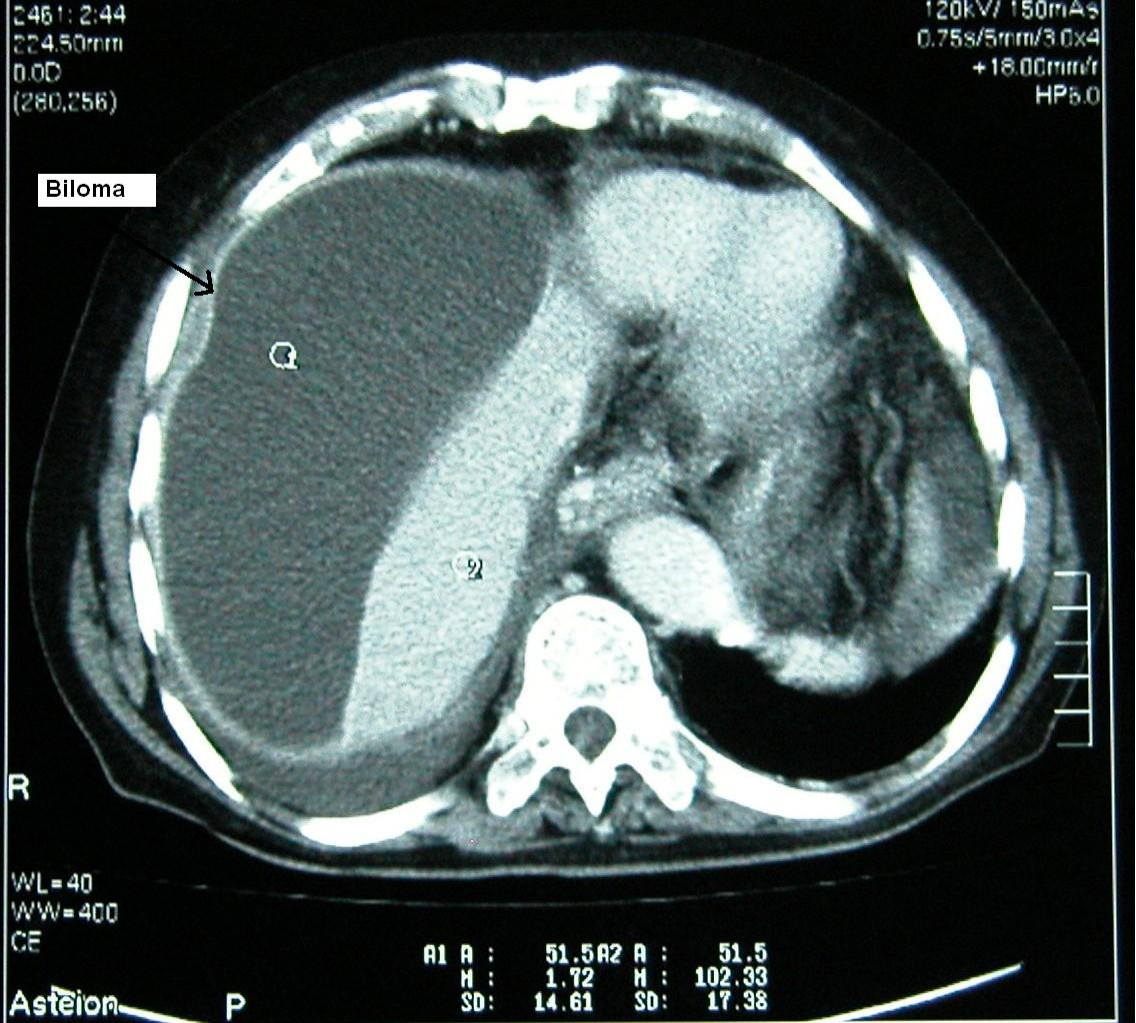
Initial abdominal CT demonstrating a large right hepatic subcapsular collection.
